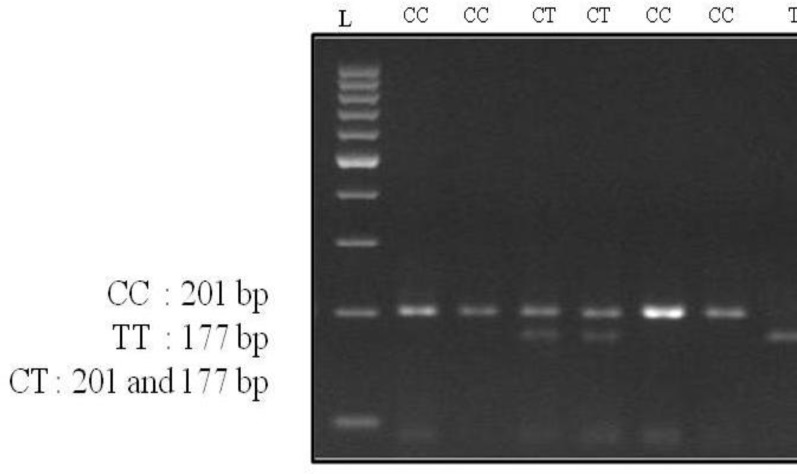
Agarose gel (2.5%) electrophoresis of HinfI digested PCR products. 100 bp DNA ladder (Fermentas) was used as marker of DNA fragment sizes. The CC genotype was confirmed by a single band of 201 bp, the TT genotype indicated by a single band of 177 bp, and; CT genotype by two bands of 201 bp and 177 bp
